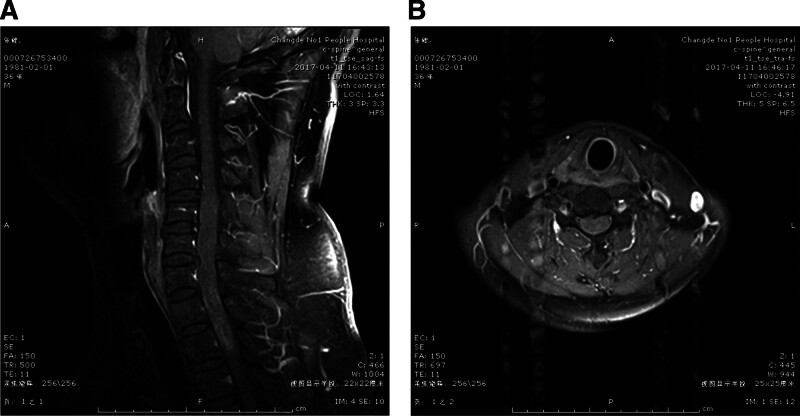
Diagnostic MRI images for the intramedullary ependymoma (C5-to-T2, 5 segments). (A, B) The sagittal and axial T1W enhanced MRI images reveal the intramedullary space-occupying mass in the cervical 5 to thoracic 2 spinal cords, which shows uniform moderate strengthening signal and cavities. C5 = 5th cervical vertebrae, MRI = magnetic resonance imaging, T2 = 2nd thoracic vertebrae.
